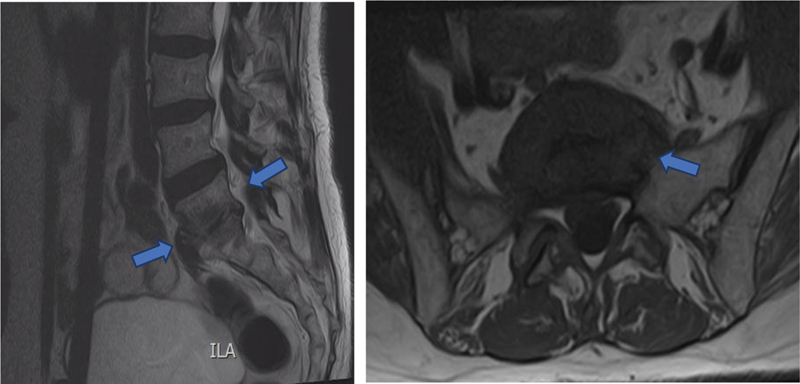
Magnetic resonance imaging after treatment – Resolution of the inflammatory peridural component with post-infection ankylosis signs of L5-S1.
